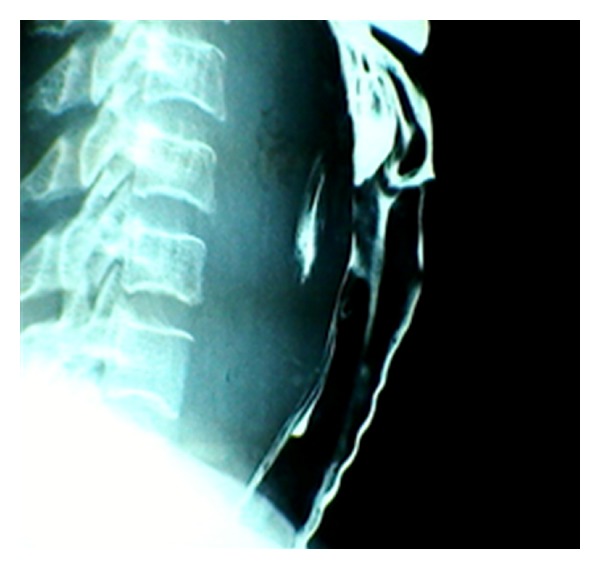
Lateral neck radiographic image (barium swallow study) of a patient who developed retropharyngeal abscess secondary to fish bone ingestion trauma.
